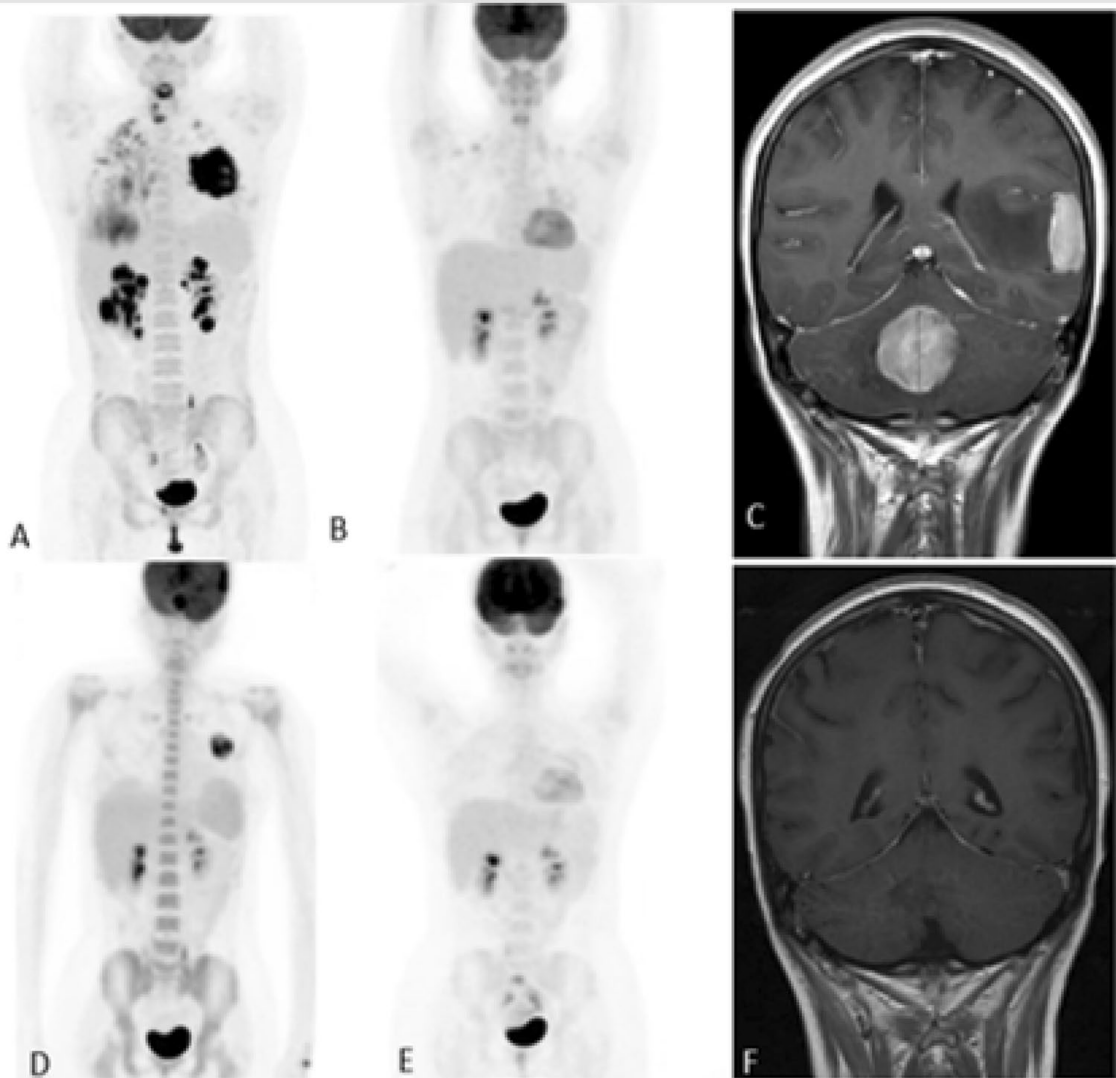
A Lymphoma involving the mediastinum, liver, with possible involvement of the kidney, and lung parenchyma. B End of therapy imaging showing complete resolution of the previously involved areas. C Left temporal dural based tumor and vermian tumor along the fourth ventricular margin, with surrounding vasogenic edema and resultant mass effect. D New hypermetabolic activity seen at the site of the initial mediastinal mass. Two new FDG avid CNS lesions. E Pretransplant PET scan showing complete resolution of the previously involved areas. F Pretransplant brain MRI showing complete resolution of the previously involved areas
